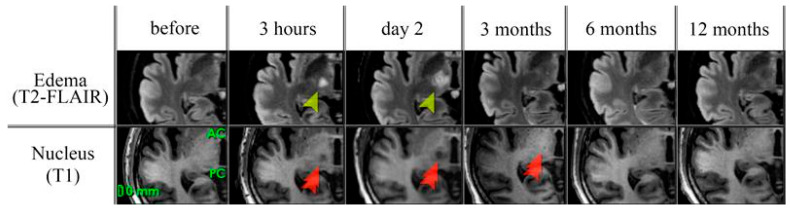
MRI follow-up before and after MRgFUS. Edema (yellow arowhead) was assessed on T2-FLAIR images, the nucleus of destruction (red double arowhead) was assessed on T1 images. The changes within VIM area were visible only at 3 h and 2 days after MRgFUS.
